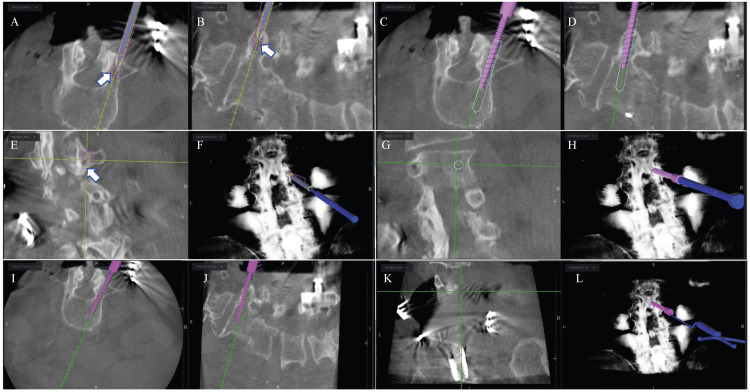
Intraoperative neuronavigation showing real-time virtual screw placement converting removed cortical screws to pedicle screws. Top row: Planning the entry point of the traditional pedicle screw. The white arrow points to the location of the previous cortical screw (hypodense). Axial representation (A) for the entry point of the virtual screw starts lateral to the entry of the cortical screw. Sagittal representation (B) shows the trajectory of the pedicle screw to be parallel to the superior endplate. Coronal representation (C) for the entry point of the virtual screw starts superior to the entry of the prior cortical screw. X-ray representation of the pedicle screw trajectory is shown in image D. Middle row: Screw advancement through the pedicle in the axial frame (E), parallel to the superior endplate in sagittal frame (F), in the coronal frame (G), and an X-ray representation (H). Bottom row: Final position of the pedicle screw in the axial frame (I), sagittal frame (J), coronal frame (K), and X-ray representation (L).
